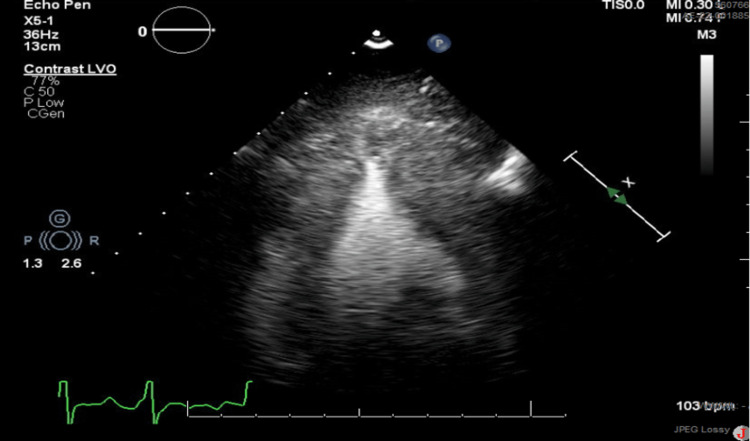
Echocardiography of the Patient’s Heart Left ventricular echocardiography shows prominent apical hypertrophy and narrowing of the left ventricular cavity, resulting in a “spade” morphology.
